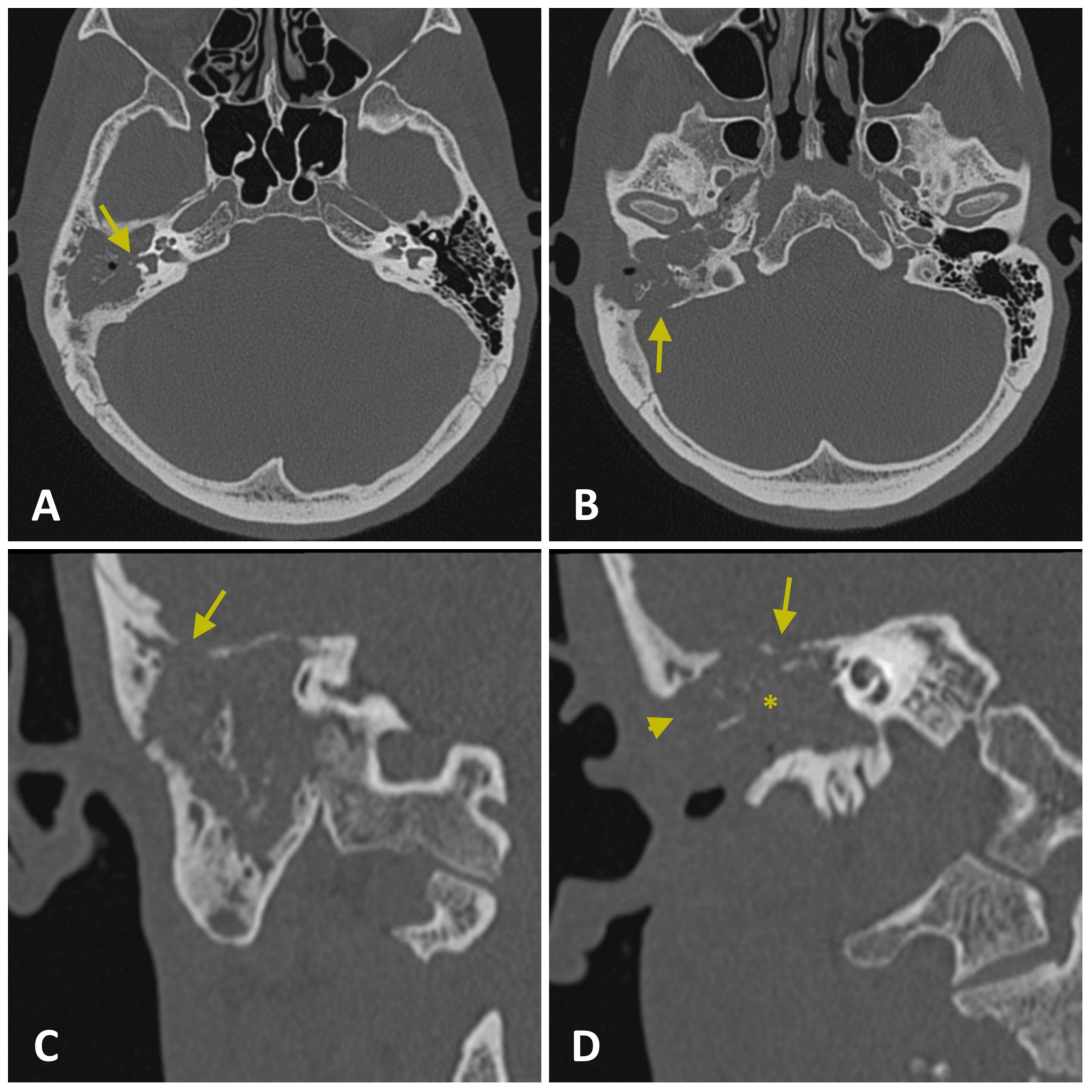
HRCT of the temporal bone detects coalescent mastoiditis with extensive bone erosion and bone sequestration on the right, mastoid cavity occupied by soft tissue density. Axial section showed (A) erosion of the lateral semicircular canal (arrow) and dehiscence of the tympanic portion of the nerve facial, (B) and with sigmoid sinus dehiscence (arrow). The coronal section showed (C) discontinuity of the middle fossa (arrow), (D) discontinuity of the tympanic tegmen (arrow), erosion of the external auditory canal (asterisk), and erosion of the cortical bone of the mastoid (arrowhead). HRCT: high-resolution computed tomography
